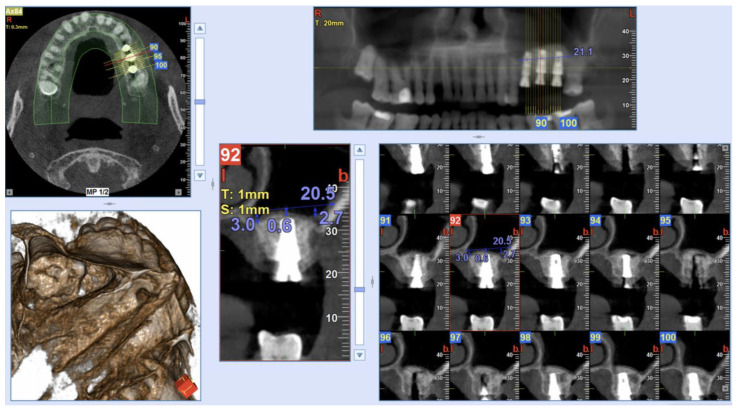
Post-operative CBCT scan after the surgical procedure and implant positioning. Measurements were taken on implants placed in 26 and 27 implant sites (Sinus-Plant implants).
